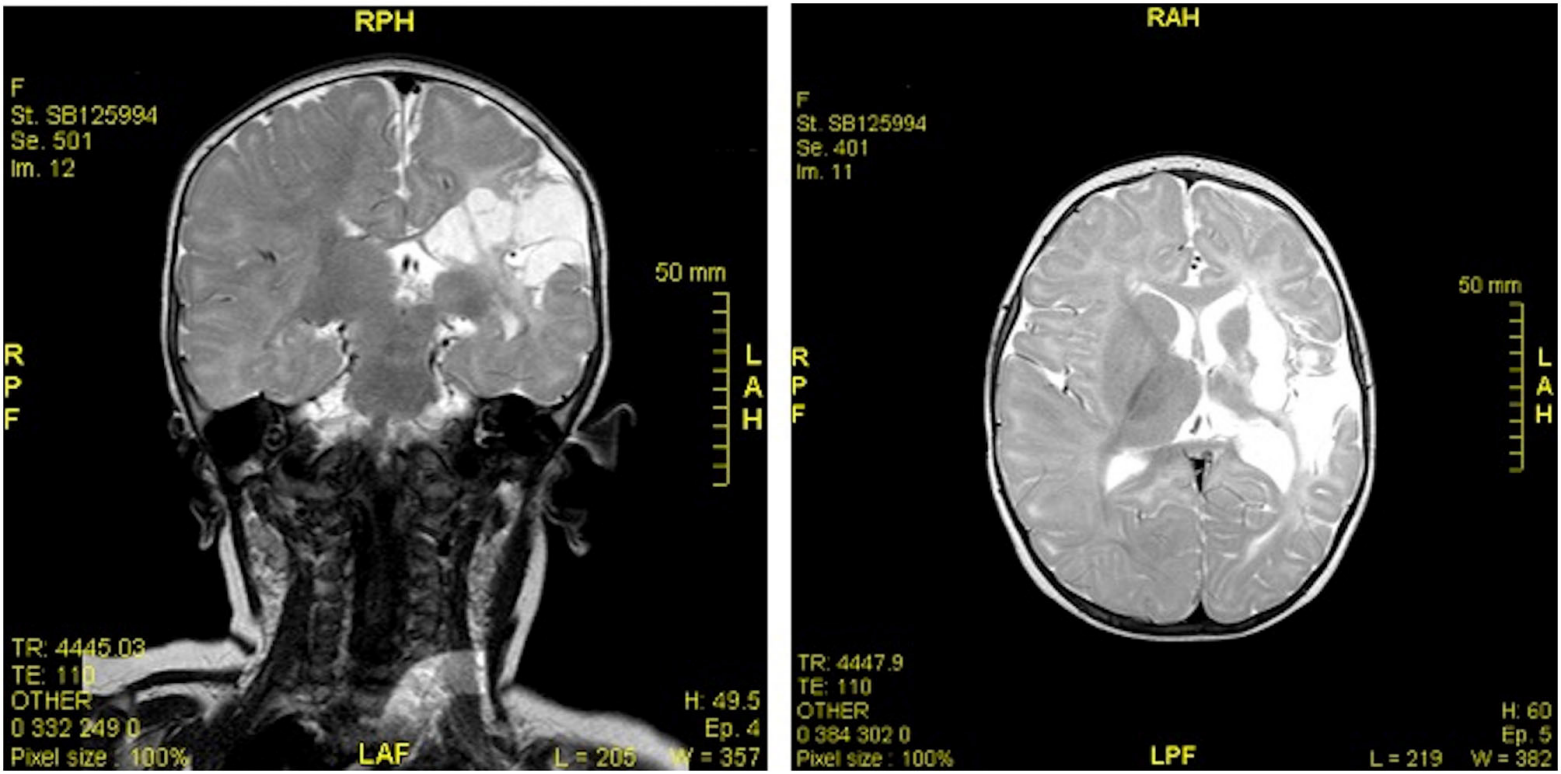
MR imaging (coronal and axial sections) showed ex-vacuo left ventricular dilatation and a cortico-subcortical fronto-temporal-parietal left malacia extending to the ipsilateral capsular regions and to the basal ganglia, without the involvement of the head of the caudate nucleus or the thalamus.
